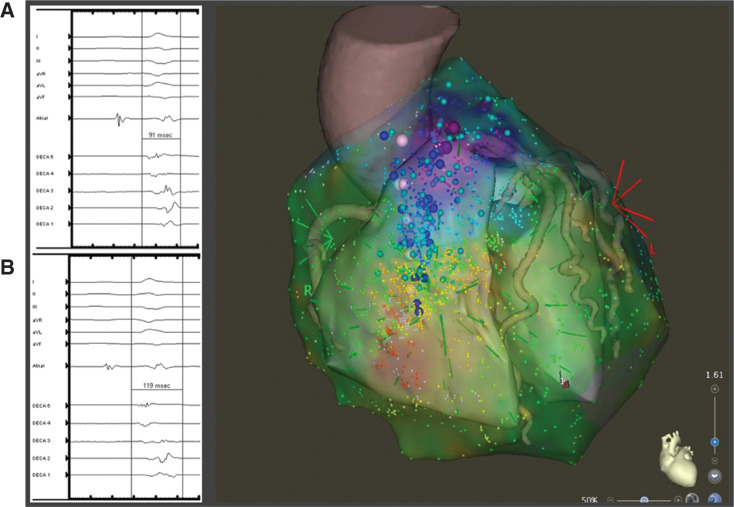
Integration of the epicardial activation map with a CARTOSOUND^®^ image and 3D CT scan with RVOT electrograms before (A) and after (B) pericardial warm saline infusion. The electrogram became more delayed and fractionated after warm saline infusion. The duration of the electrogram was measured from the beginning of the QRS complex.
